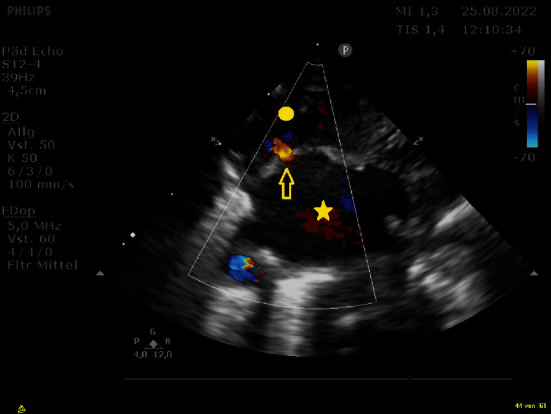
Echocardiography of atrial anatomy of the patient showing a severely dilated left atrium (star), a restrictive atrial septal defect (arrow), and a small right atrium (dot).
